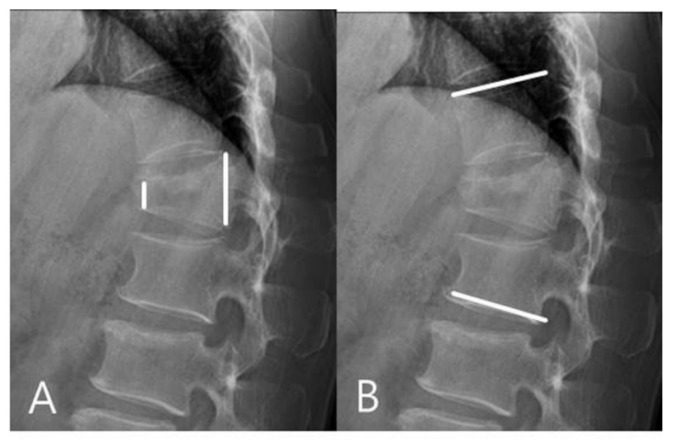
(A) Sagittal index (SI) was calculated by the ratio of the heights of the anterior and posterior vertebral wall. (B) Regional kyphosis angle (RKA) was measured on lateral radiographs using the Cobb method. RKA between the superior endplate of the vertebra above the fractured vertebra and the inferior endplate of the vertebra below the fractured vertebra were measured.
